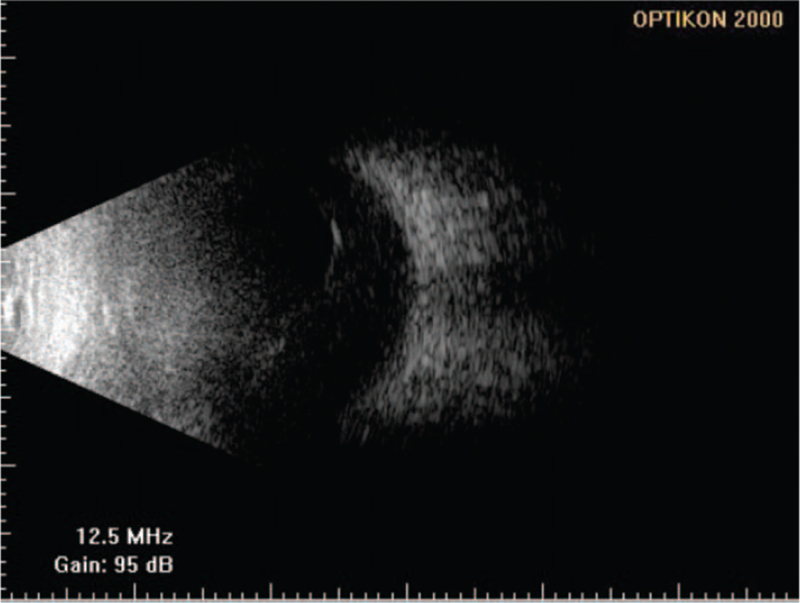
B-scan ultrasonography of the left eye. Mild vitreous opacity.
